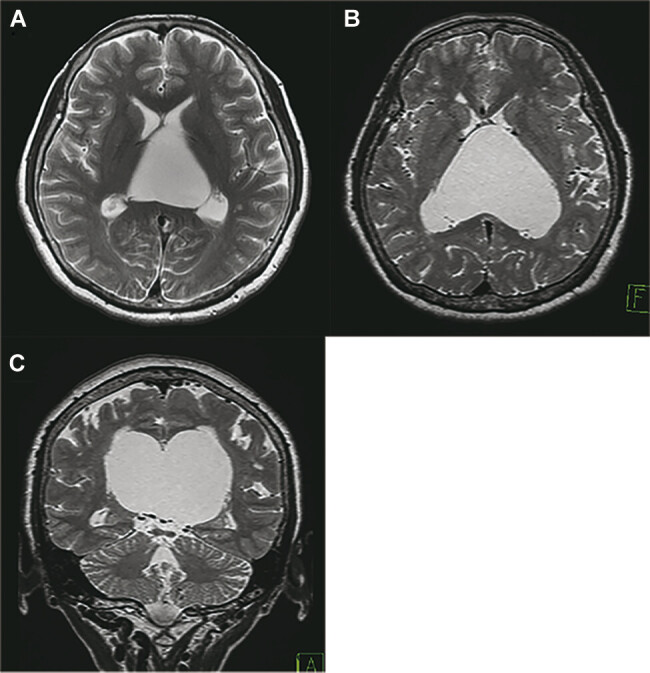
Serial head MRI before surgery. (A) Head MRI taken 8 years before admission. A cyst in the velum interpositum is revealed. (B, C) MRI taken before surgery. The cyst expands, and the lateral ventricle decreases in size. Internal cerebral veins (ICVs) are located at the bottom of the cyst. No tightness of sulci is observed. MRI, magnetic resonance imaging.
